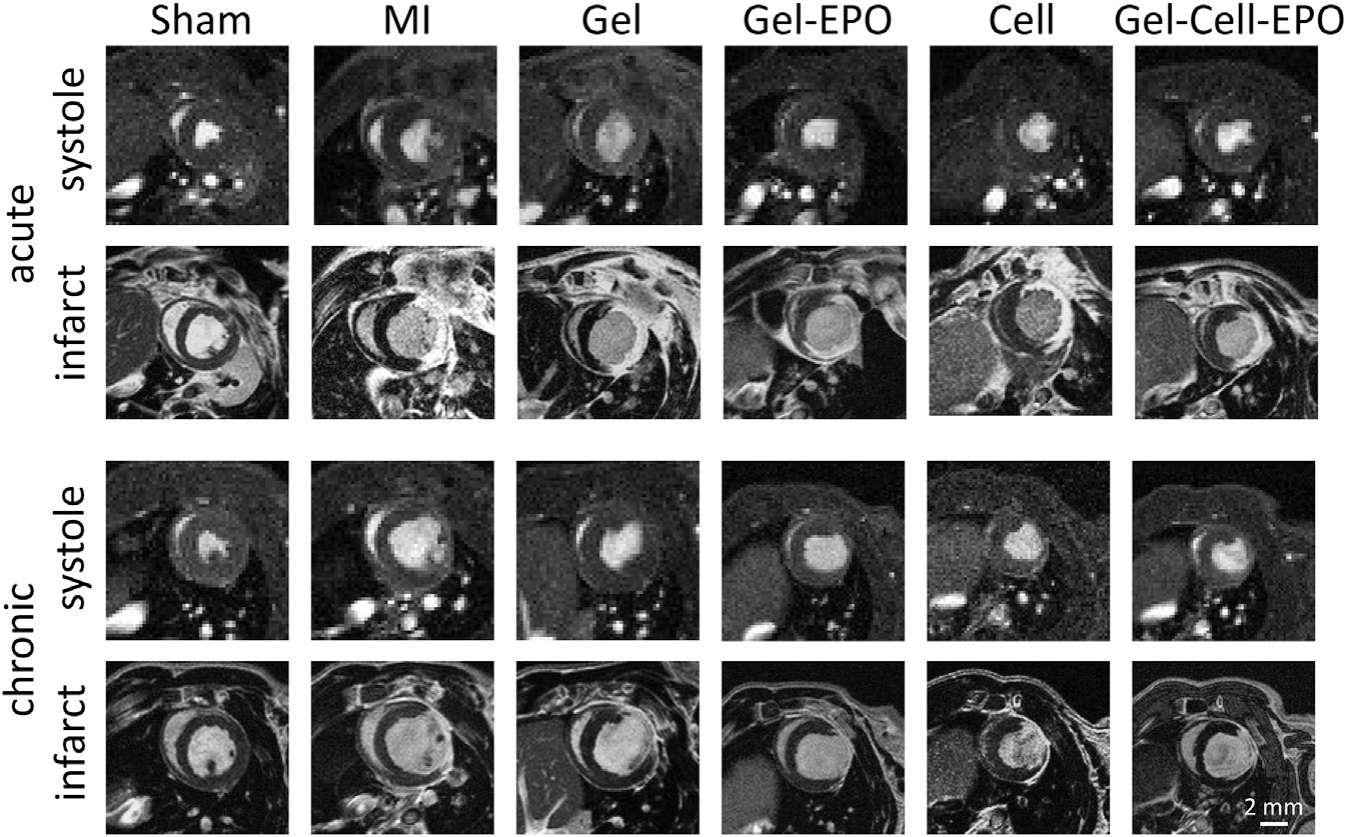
MRI Characterization of Cardiac Structure, Function, and Viability Acutely and Chronically after MI The systolic image shows the end systolic frame of a mid-ventricular cine-MR image. The infarct image shows a late gadolinium-enhanced MRI of the hyper-enhanced infarct region at the same position as the cine-MR image.
